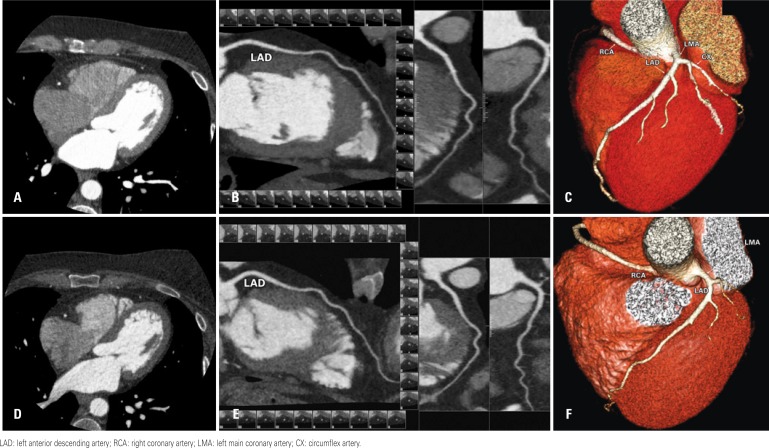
Comparison between two coronary computed tomographic angiography examinations of the same patient, the first without AIDR-3D (Figures A-C) and the second with AIDR-3D (Figures D-F). The noise is different on axial computed tomographic images (Figures A and D), but the curved-MIP (Figures B and E) and 3D volume-rendering (Figures C and F) reconstructions are similar. Estimated effective radiation dose from coronary computed tomography angiography only and full examination were respectively 6.6mSv and 8.8mSv (exam without AIDR-3D), and 1.97mSv and 3.9mSv (exam with AIDR-3D)
